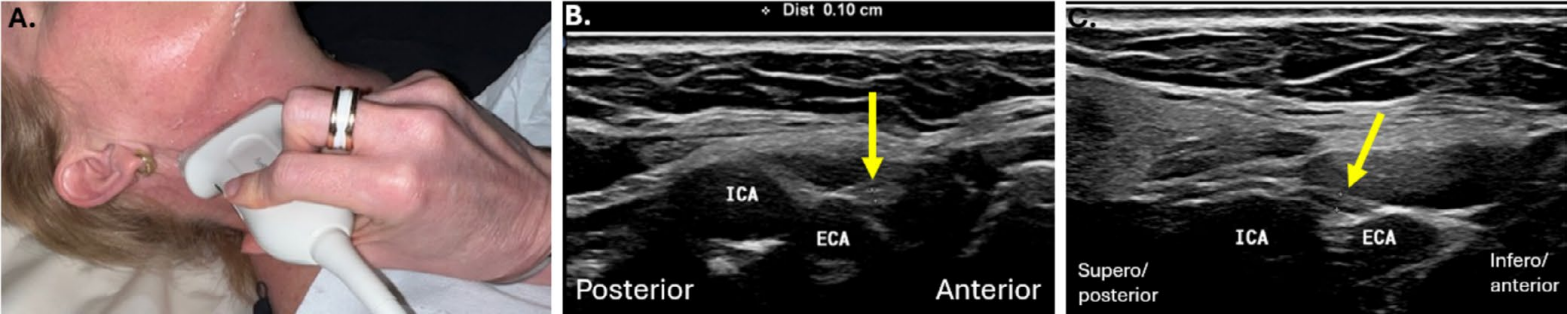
(A) Transducer positioning for imaging where the hypoglossal nerve changes from its horizontal to vertical component. Sonographic imaging of the posterior horizontal portion of the hypoglossal nerve (B) and the start of the vertical component of the hypoglossal nerve (C).
